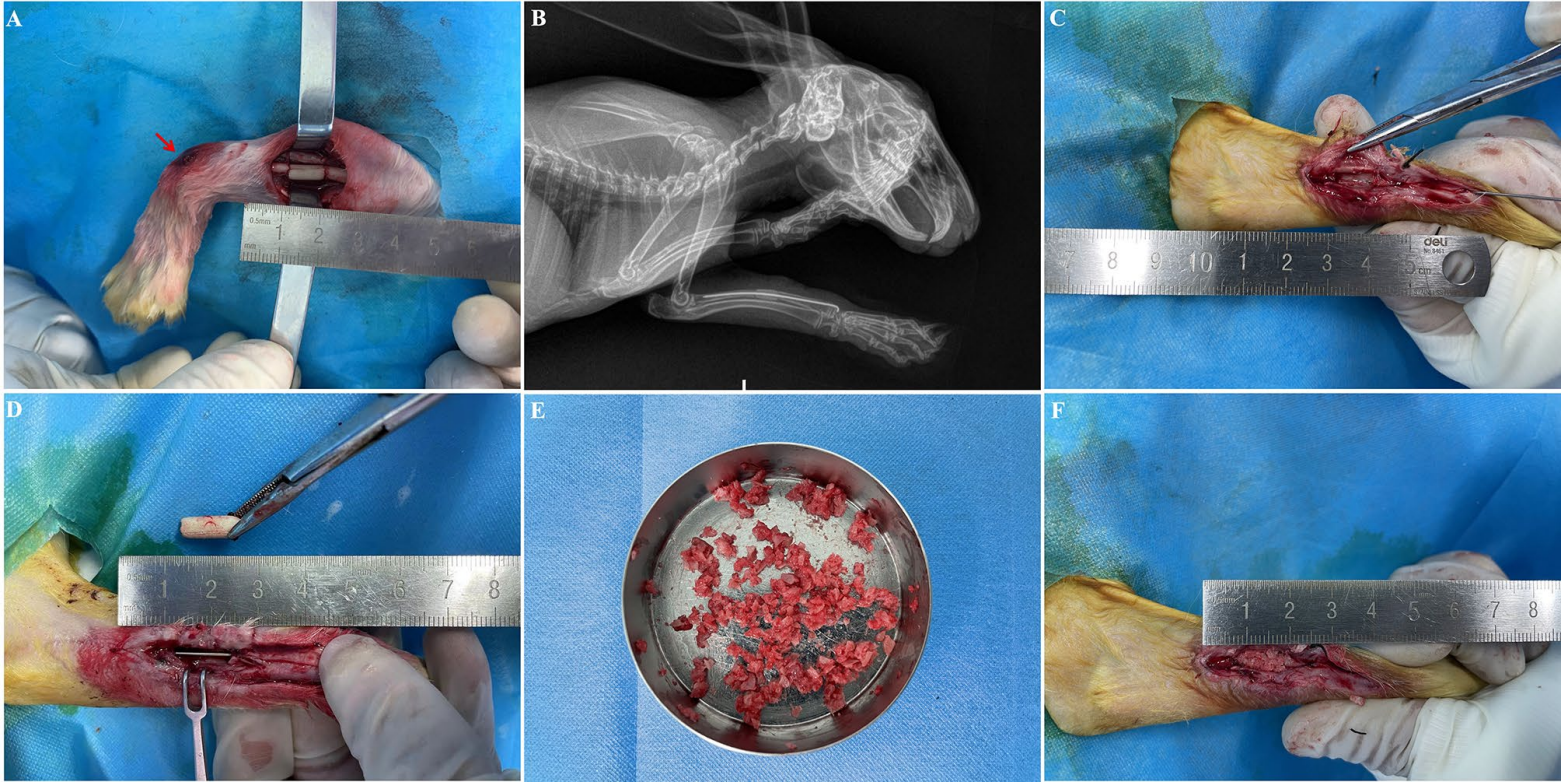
Application of the Masquelet technique. A critical bone defect (10 mm) was created at approximately the middle of the left radial shaft, and the defect was inserted with a cylindrical PMMA spacer and subsequently fixed intramedullary with a retrograde Kirschner’s wire; the red arrow indicated the internal fixation point, and the Kirschner’s wire was curved by a clamp at this puncture spot by a clamp (A). Postoperative X-ray revealed the good location and rigid internal fixation of the PMMA spacer (B). Four weeks after PMMA implantation, the pre-curved tail of the Kirschner’s wire was exposed and pulled out partly for the subsequent removal of the PMMA (C). The Kirschner’s wire was reinserted into the cavity of the radius (D). Allogeneic iliac bone was harvested and cut into pieces for further bone grafting (E). An adequate volume of graft material was implanted into the bone defect (F).
